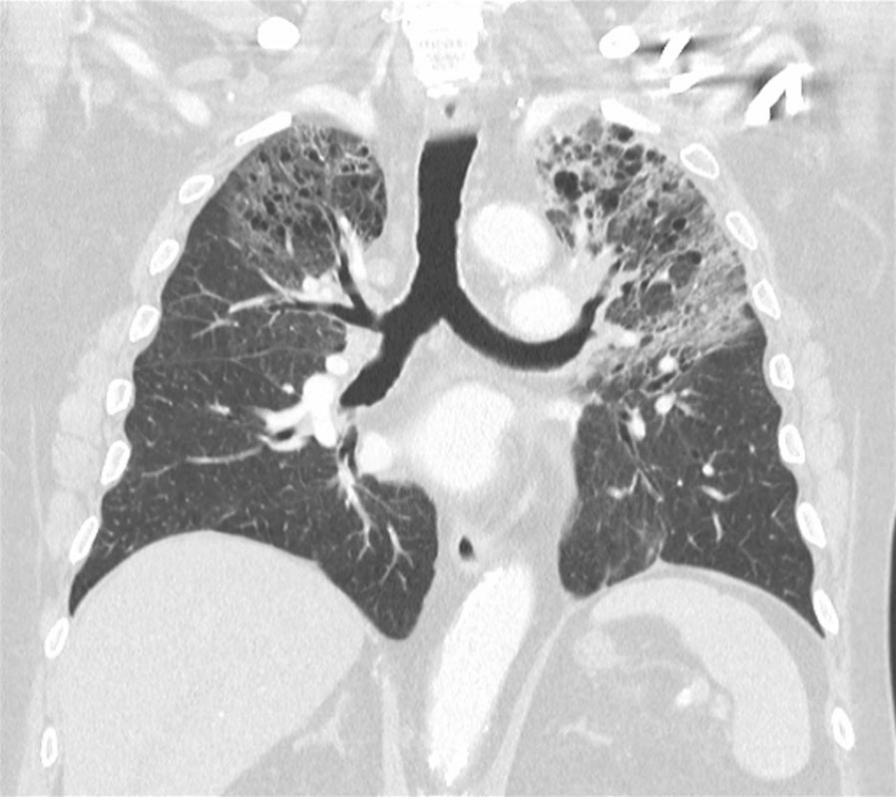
Case 3—CT chest after immunotherapy given revealing signs of pneumonitis, diffuse peribronchial ground-glass changes. There is decrease in size of lung cancer
